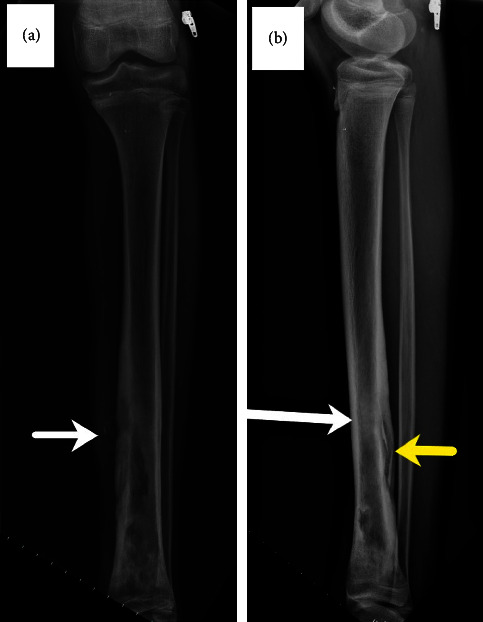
Plain radiographs of the left tibia showed osseous destruction throughout on (a) anteroposterior view and (b) lateral view with the sinus tract (white arrow), sequestrum, and surrounding involucrum (yellow arrow).
